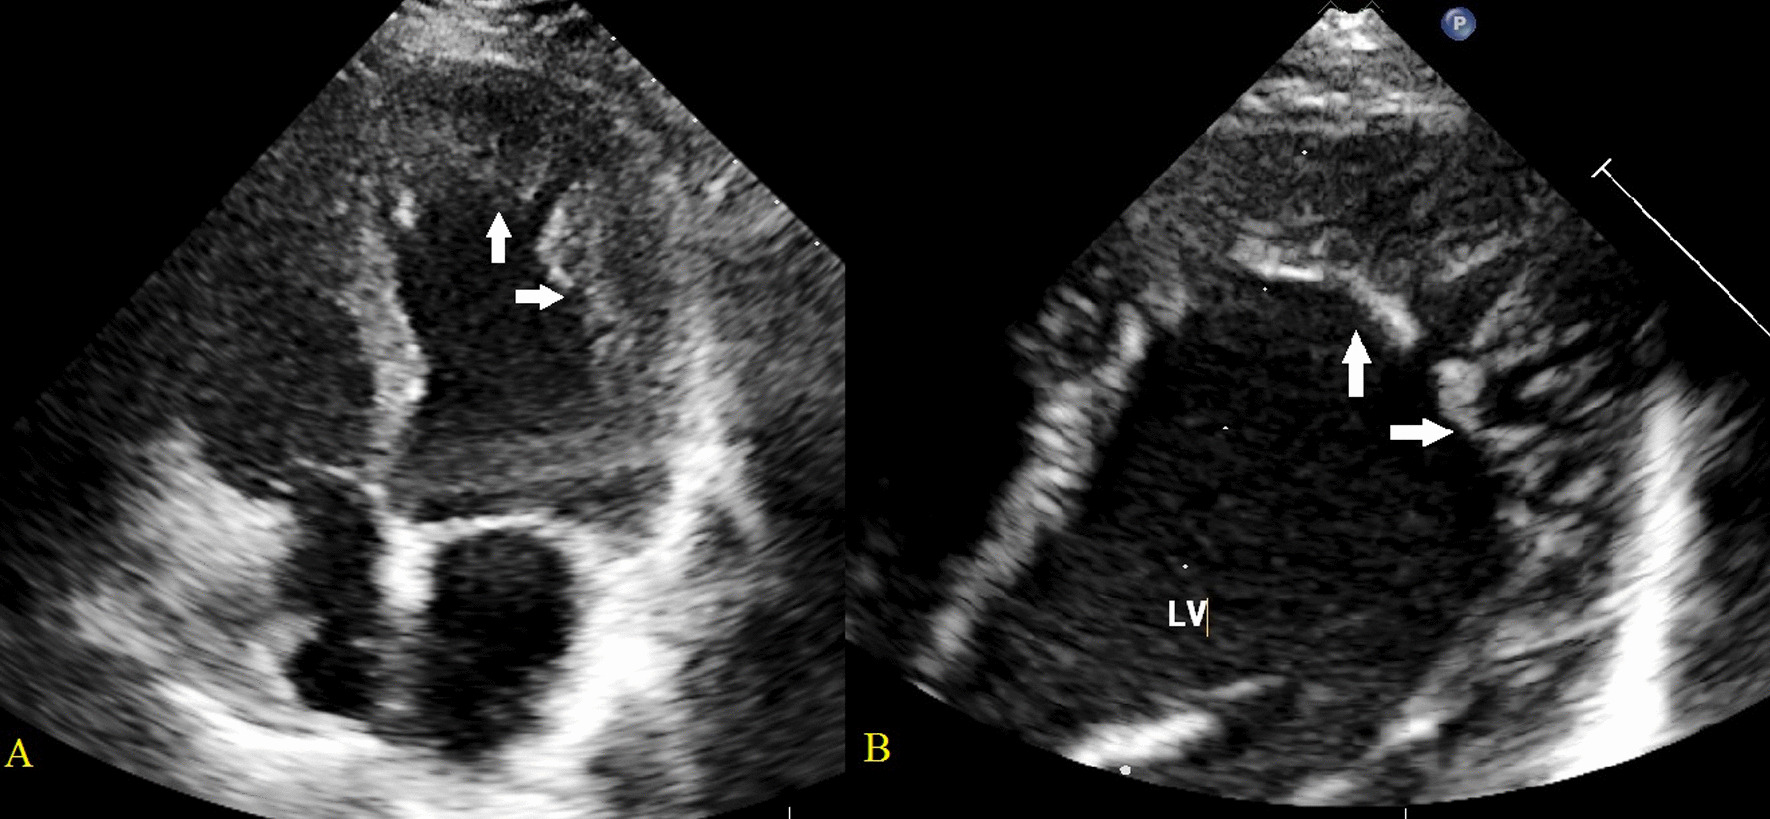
Transthoracic echocardiographic images. Apical 4-chamber views demonstrating LVNC with excessive trabeculations (arrow) in the grandmother (A) and older sister of the fetus (B)
